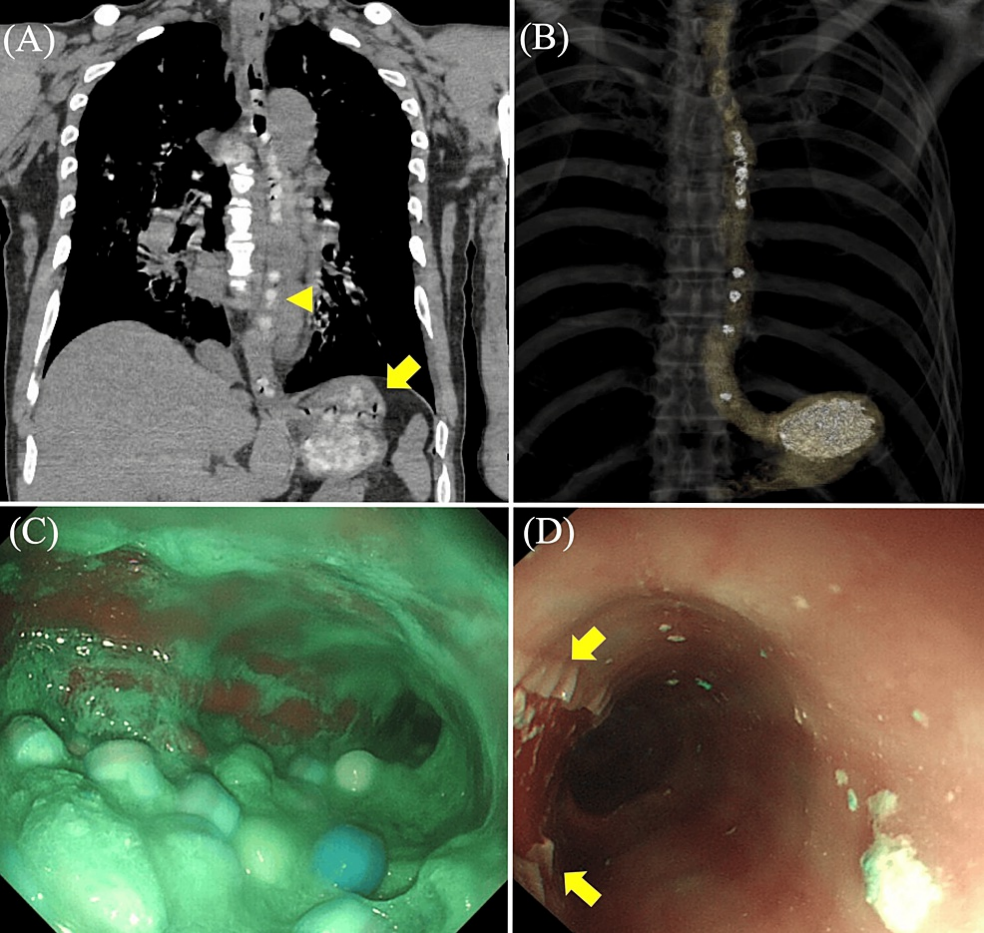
CT and EGD (A) Axial plain CT of the chest showed high attenuation areas in the esophagus (yellow arrowhead) and stomach (yellow arrow). (B) Three-dimensional volume-rendered CT showed the presence of ingested tablets in the esophagus and stomach. (C) EGD showed a large amount of pharmacobezoars in the stomach. (D) During endoscopic decontamination with a collection net, an esophageal tear occurred due to a collection net (yellow arrow). CT, computed tomography; EGD, esophagogastroduodenoscopy
